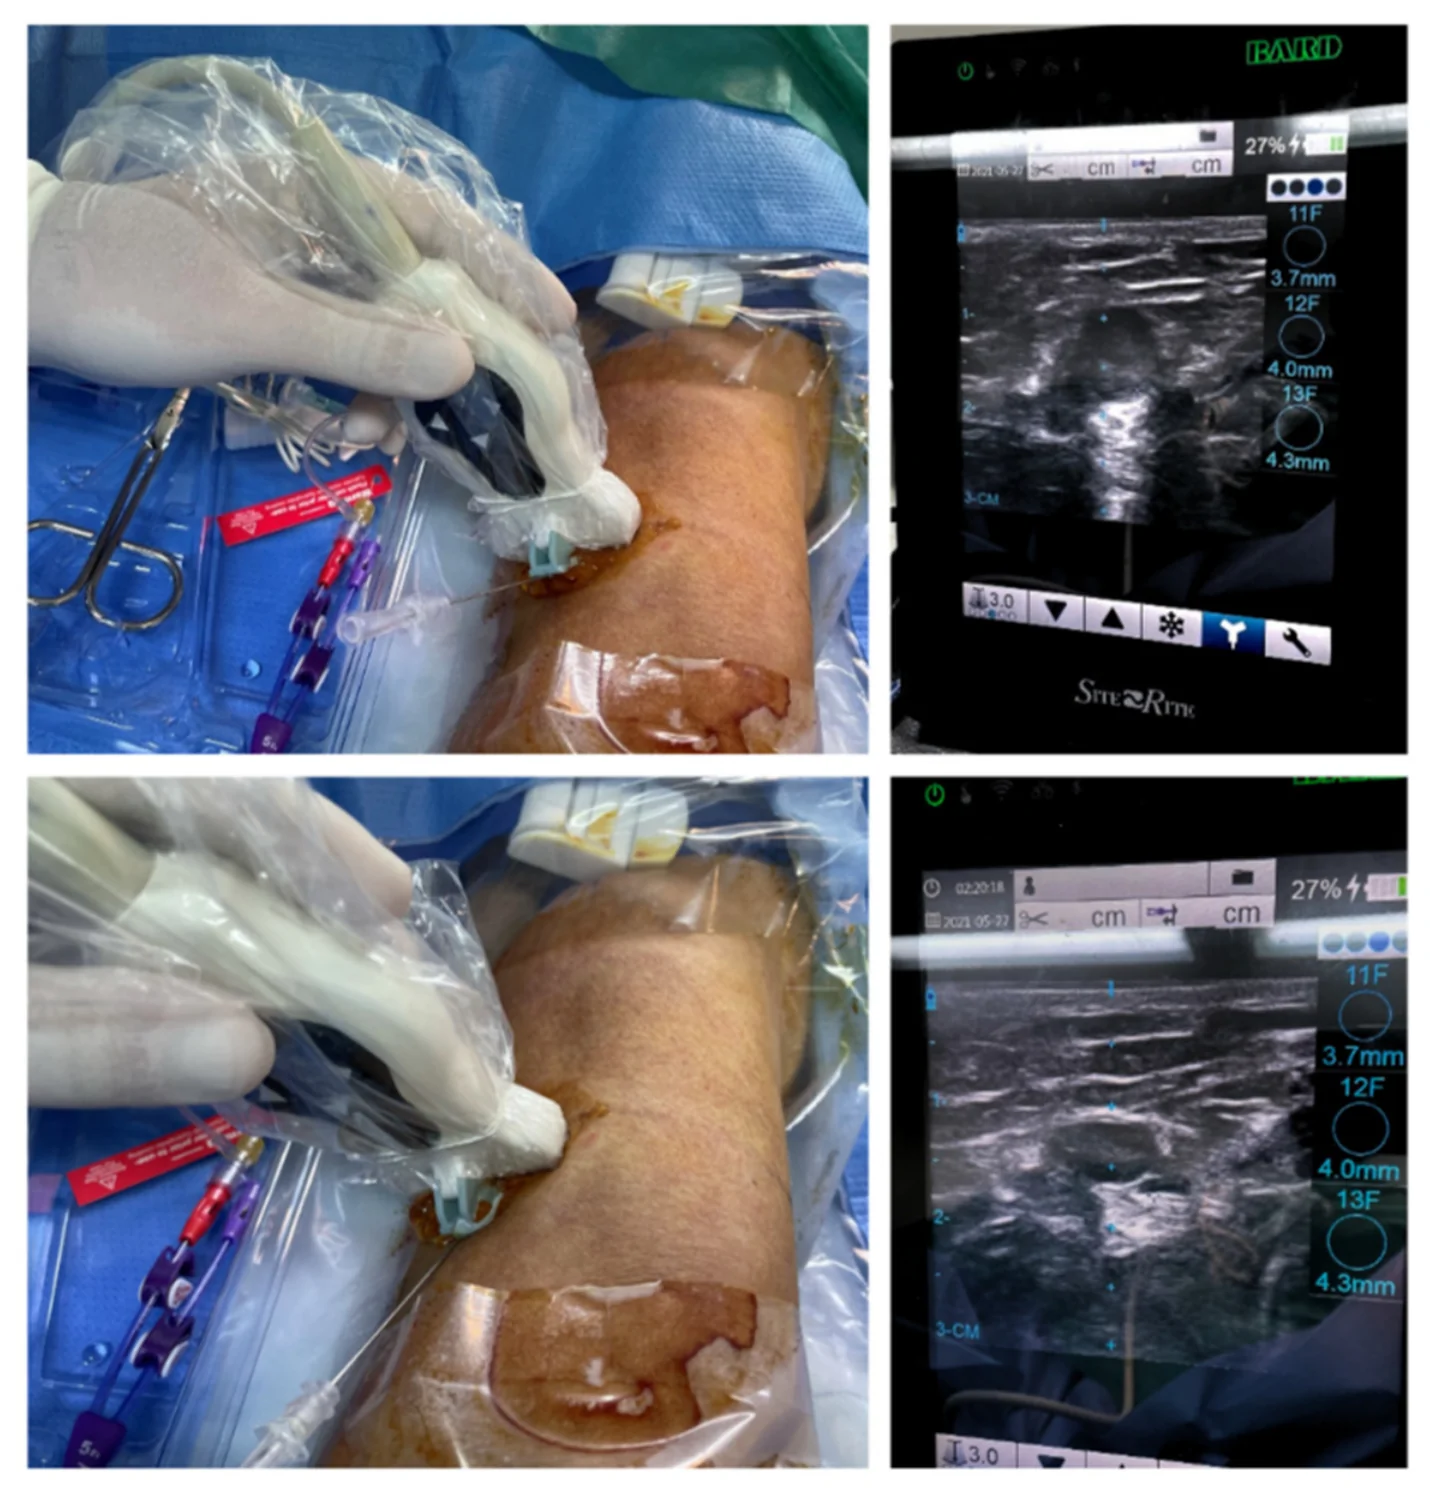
Tilting of the ultrasound transducer to facilitate cannulation of superficial veins.
